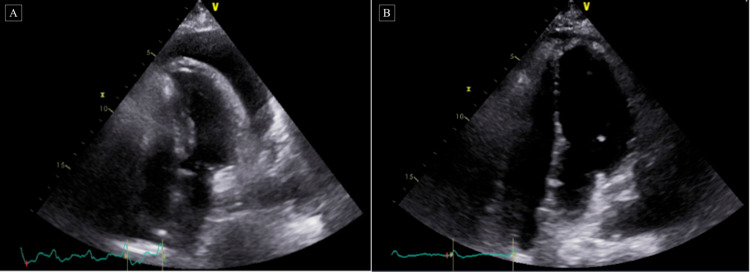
Echocardiograms before and after corticosteroids therapy A: Limited echocardiogram to evaluate for pericardial effusion. Large circumferential pericardial effusion with the diastolic collapse of the right ventricle and respiratory variation of mitral inflow consistent with cardiac tamponade. B: Limited follow up echocardiogram following steroids therapy after pericardial window. Small pericardial effusion essentially resolved compared to prior echocardiogram.
